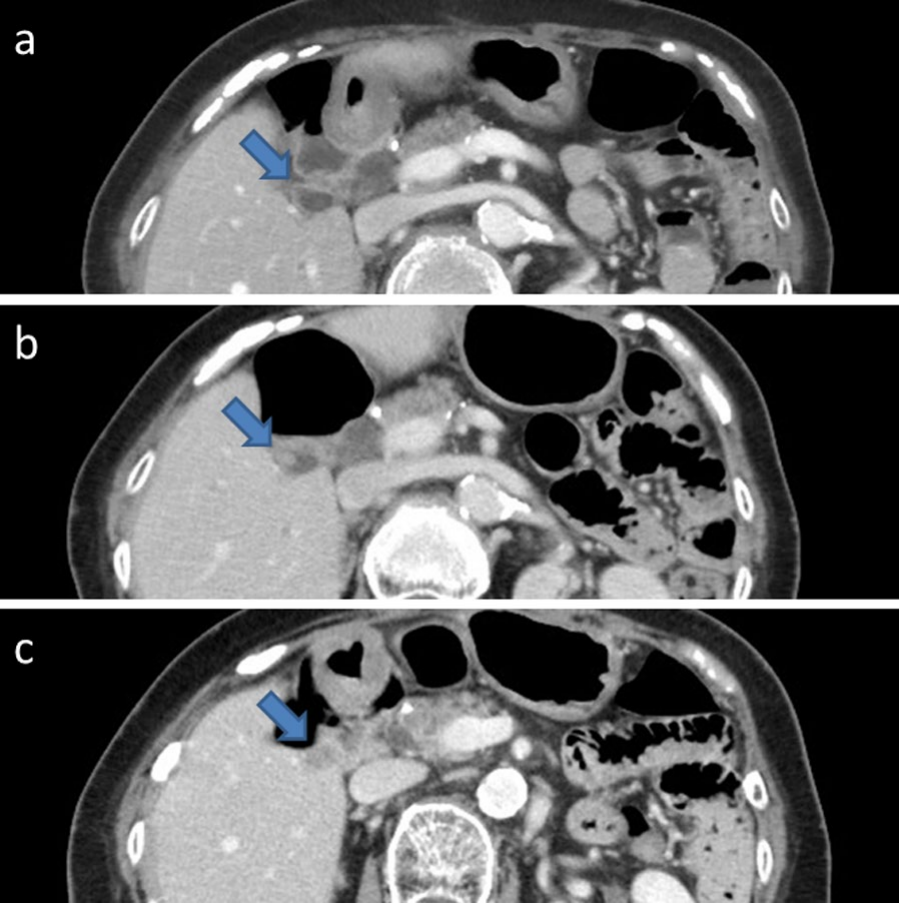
Contrast-enhanced computed tomography (CECT) findings. a Preoperative CECT for sigmoid colon cancer. The cystic remnant gallbladder without a nodule is visible (arrow). b CECT performed 6 months after the colon surgery. A 5-mm small nodule is visible in the remnant gallbladder (arrow). c CECT performed 9 months after the colon surgery. A 10-mm enhanced nodule is visible in the remnant gallbladder (arrow)
